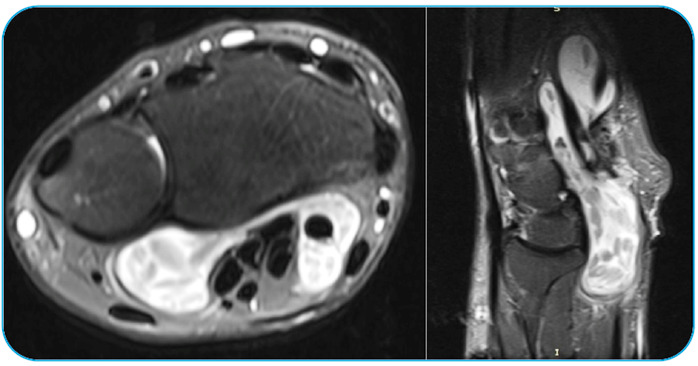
Magnetic resonance imaging of the hand and wrist displaying multiple, round, multivesicular daughter cysts occupying almost the entire volume of the mother cyst confined to the soft tissues at the flexor surface surrounding the all-flexor tendons.
